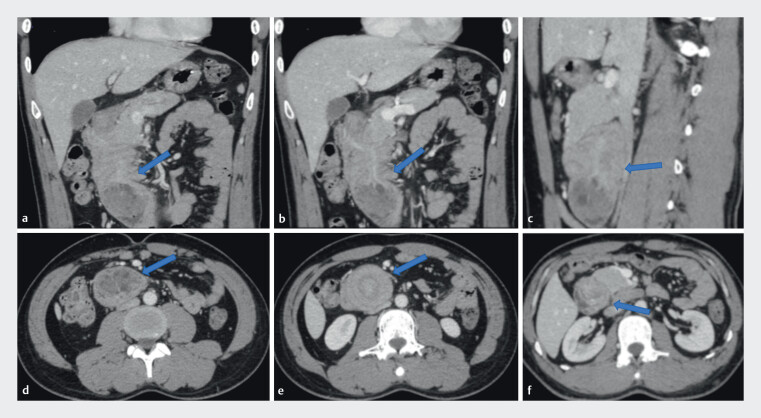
Contrast-enhanced computed tomography demonstrating a duodenal mass.
